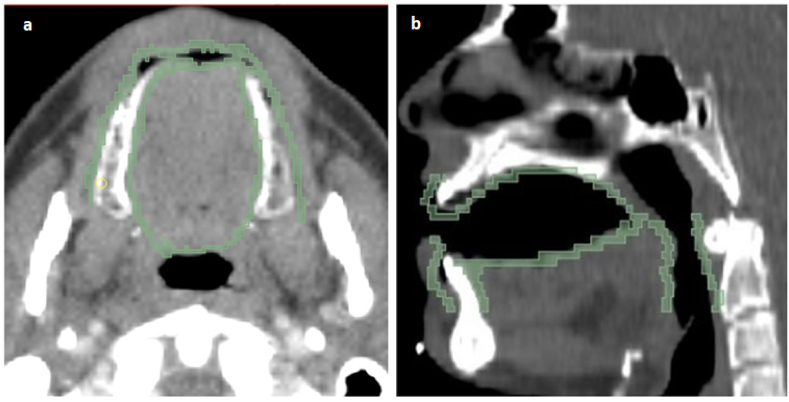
Manual segmentation of oral mucosa on CT image from a representative patient a) axial and b) sagittal view.
